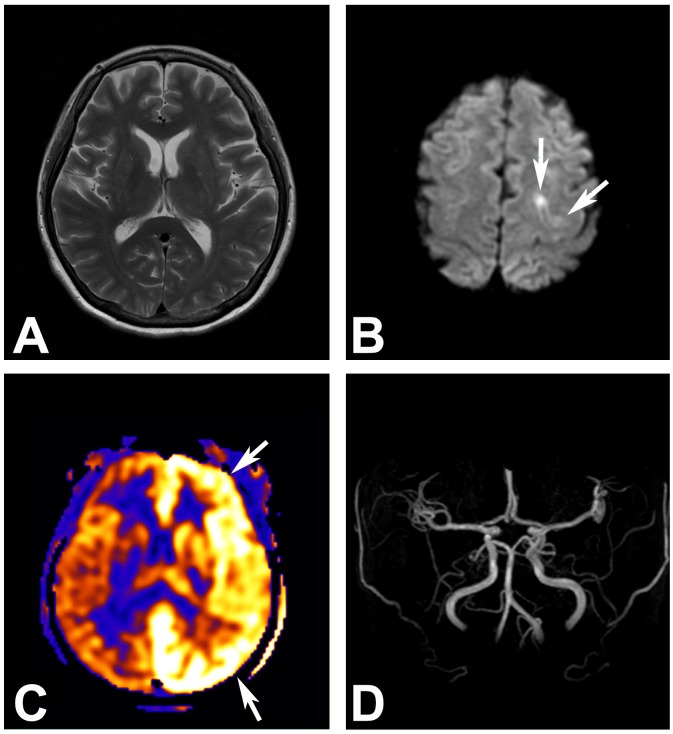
A 49-year-old woman who showed a convulsive seizure in the intensive care unit. (A) Brain parenchyma appears normal on the T2 FLAIR image. (B) Ill-defined and subtle diffusion restriction is noted at the left parietal cortex (arrows). (C) Arterial spin labeling perfusion-weighted image, however, clearly depicts hemispheric hyperperfusion (arrows) in the left cerebral hemisphere. (D) No apparent steno-occlusive lesion is found on the MR angiography.
